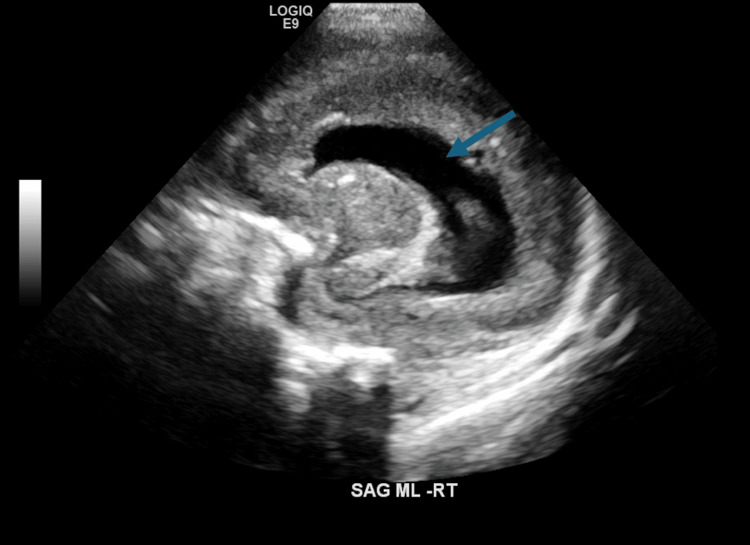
Still image from the head ultrasound on day of life 27, suggestive of an absent corpus callous (blue arrow)
